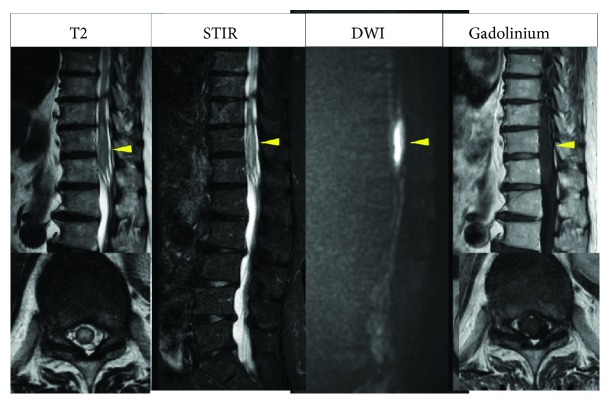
STIR and diffusion-weighted MRI distinctly showing abnormality but no lesion enhancement.
